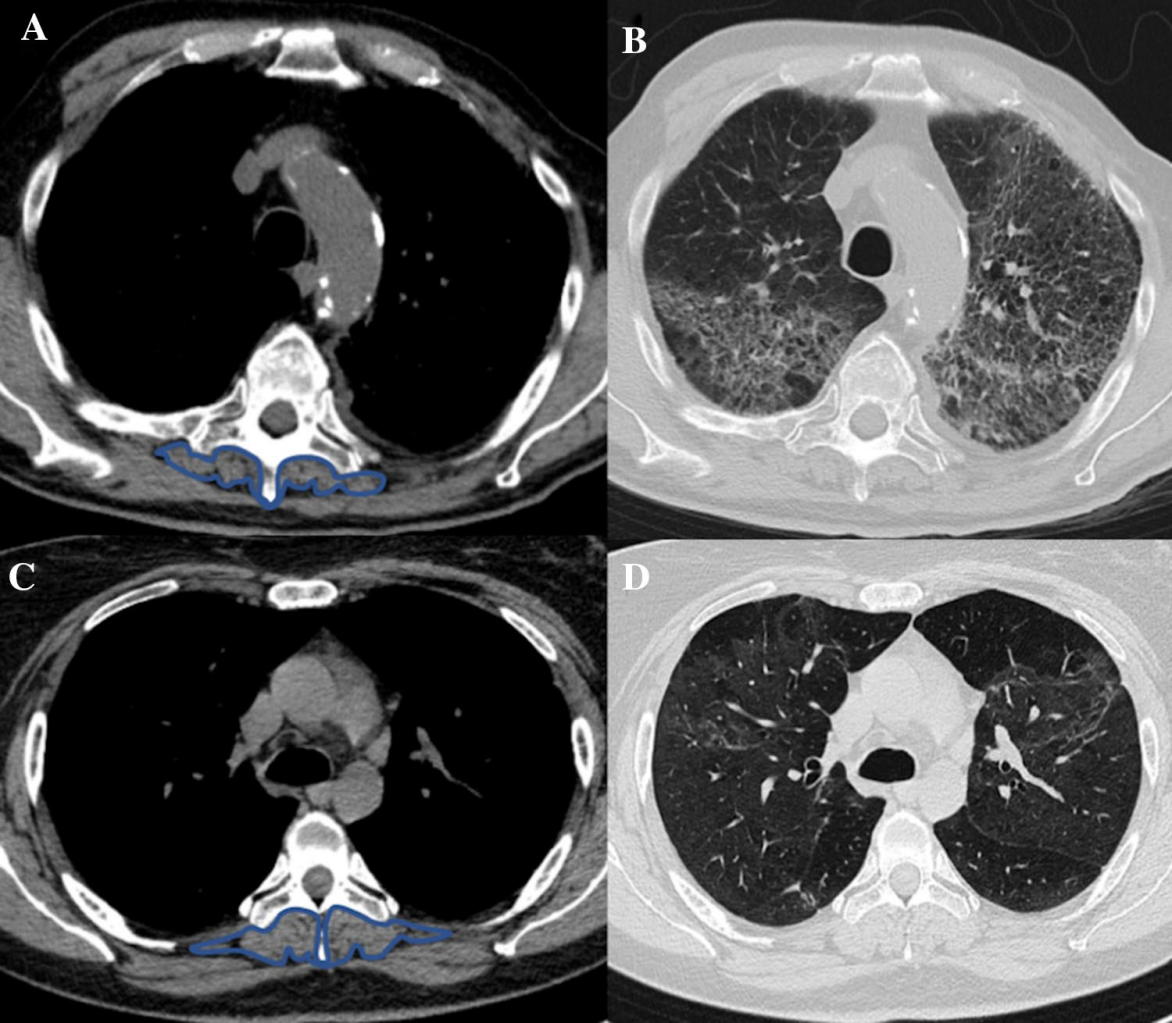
Computed tomography images of two patients affected by COVID-19 pneumonia. Images A and B refer to a sarcopenic male patient (skeletal muscle index of 42 cm^2^/m^2^) with severe parenchymal disease extent, while images C and D refer to a non-sarcopenic female patient (skeletal muscle index of 55 cm^2^/m^2^) with minimal parenchymal involvement
